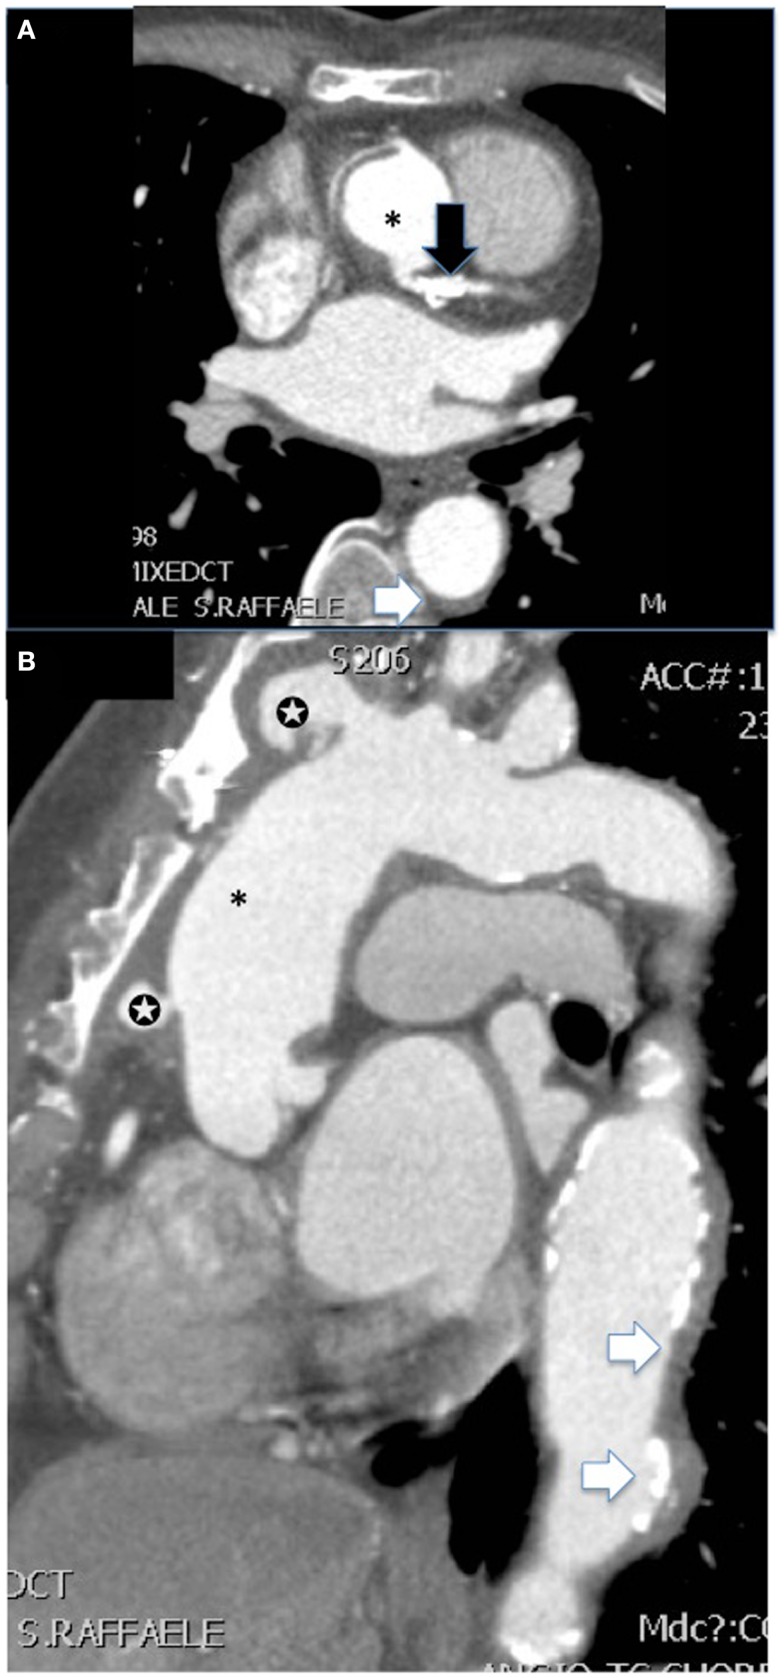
CT angiography [(A) shows axial and (B) sagittal view] of the aorta of a female patient with advanced Takayasu arteritis who underwent previous surgical repair of an ascending aorta aneurysm (*). Several features of the disease are summarized in these images: post-surgical complications such as pseudoaneurysms at the level of proximal and distal anastomosis of the vascular graft with the native aorta (white stars), extensive concentric mural thickening with calcifications of the aorta (white arrows), and presence of a stent in the main steam with a calcific stenosis (black arrow).
